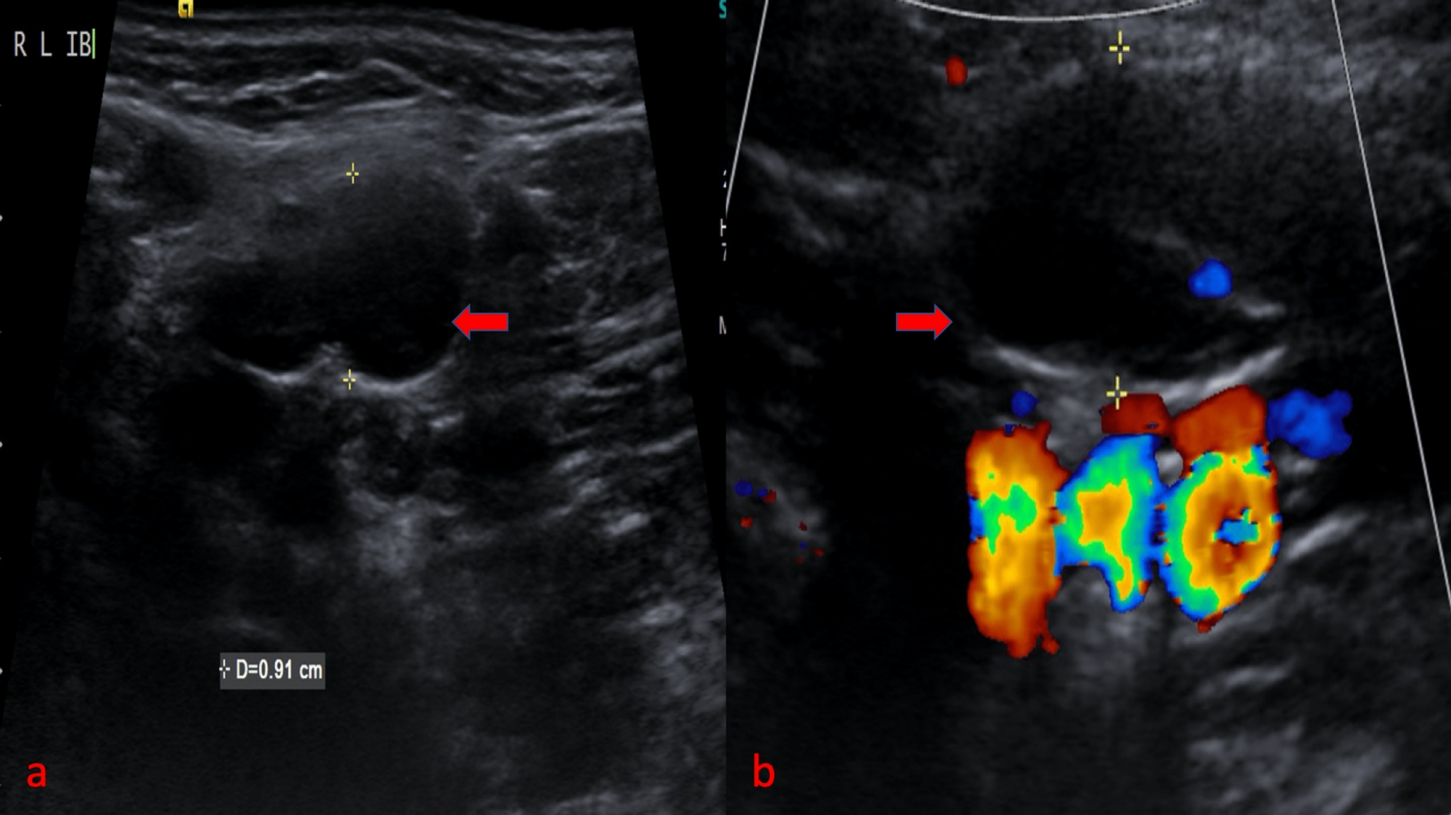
Imaging of reactive cervical lymph node: (a) BMUS shows an enlarged node with compressed hilum (red arrow), (b) CDUS shows hilar flow (red arrow) BMUS: B-mode ultrasound; CDUS: color doppler ultrasound
